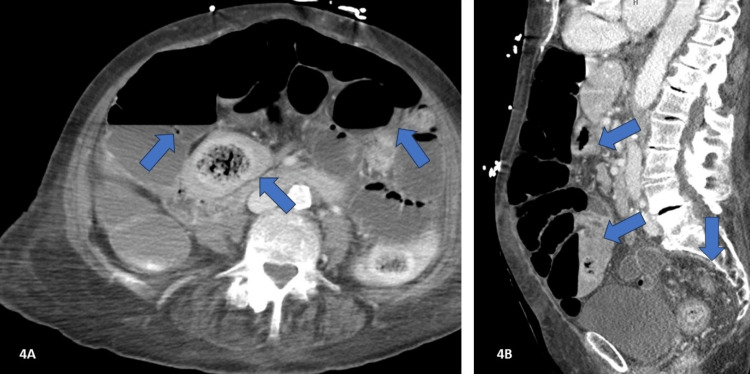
CT with contrast of the abdomen and pelvis - image 2 Impression: a large amount of stool within the colon. There is thickening and induration of the colon, compatible with colitis in the proper clinical setting. There is severe upstream dilatation of the colon and small bowel likely due to obstruction secondary to inflammation and constipation. Recommend surgical consultation. The findings are worse compared to the prior study CT: computed tomography
